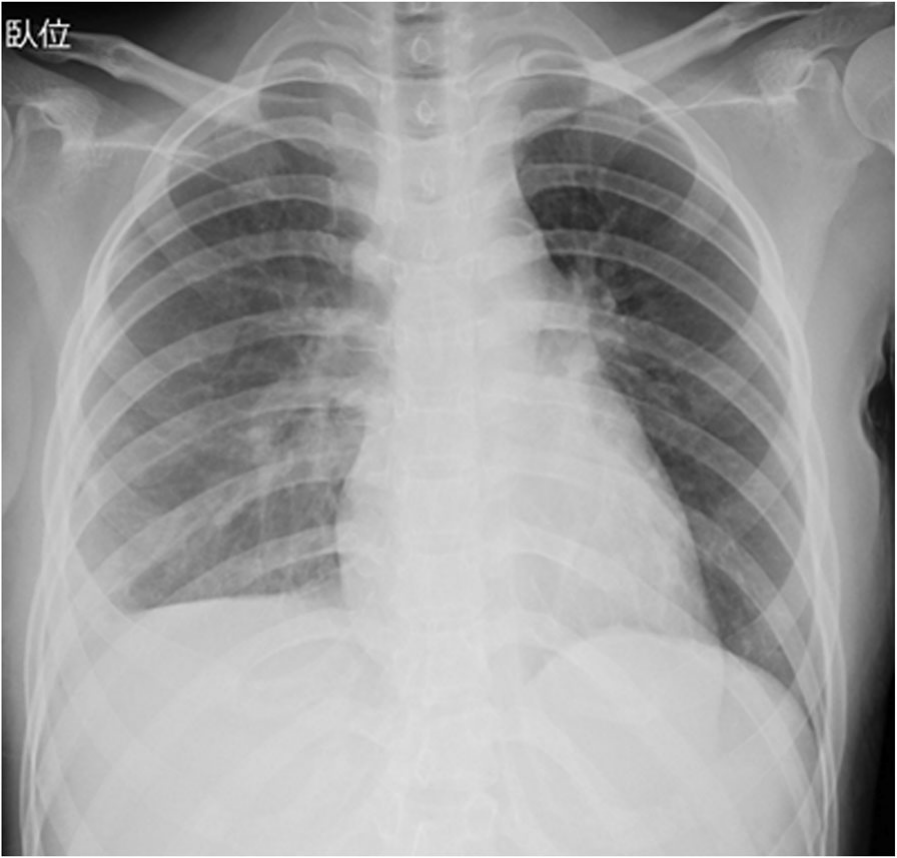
Radiograph findings from the patient’s chest. Chest radiograph showed a normal cardiac size (cardiothoracic ratio, 49%), pulmonary congestion, as well as pleural effusion in the right lung only.
